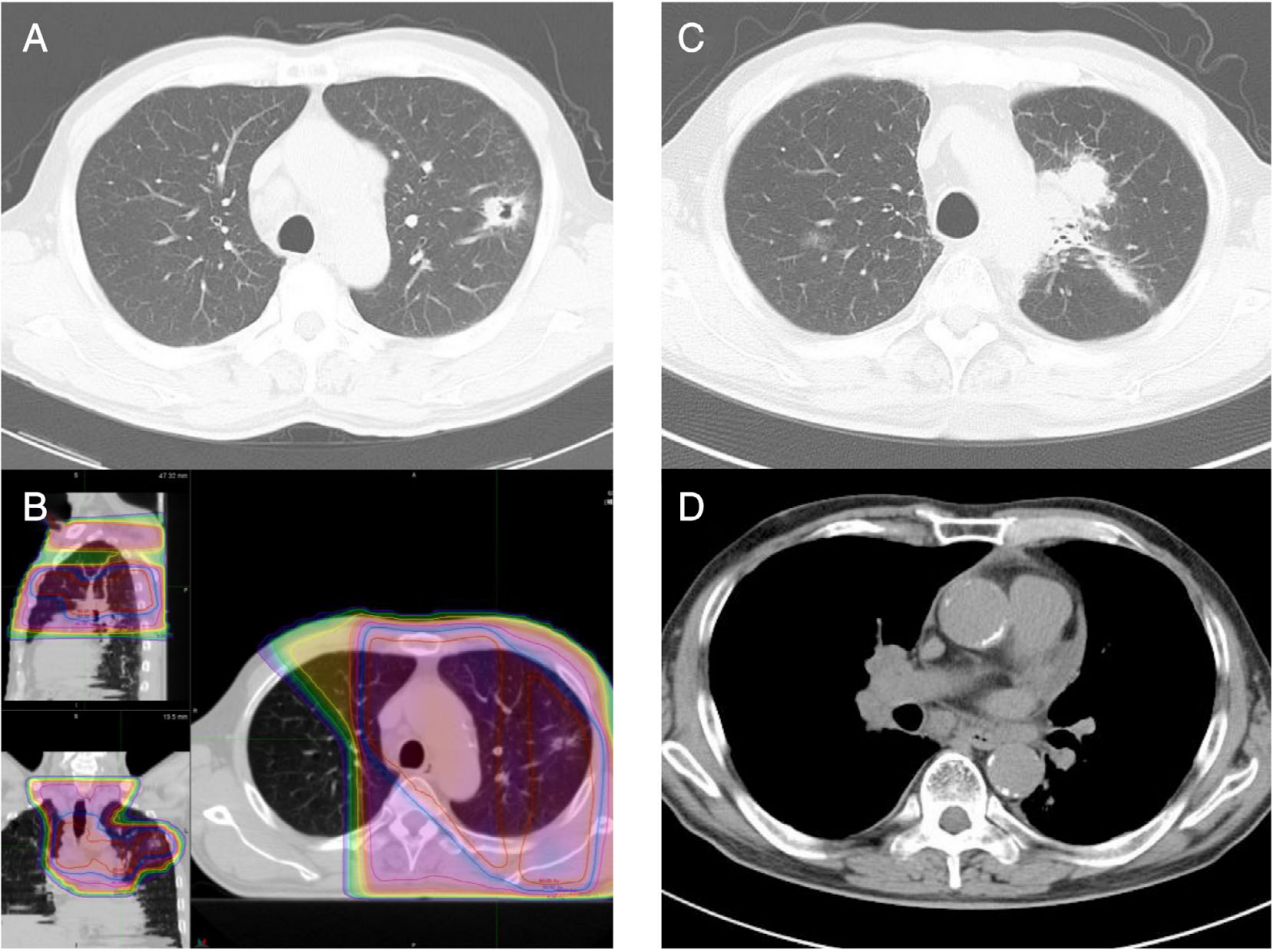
Images of case 1. (A) Thoracic computed tomography showing a pulmonary nodule with a cavity in the left upper lobe and lymph node swelling of the mediastinum at the onset of lung adenocarcinoma when the patient was 62 years. (B) Map of radiation dose distribution in radiotherapy for lung adenocarcinoma. (C) A mass seen in the left upper lobe when the patient visited the hospital for haemoptysis. (D) Swollen lymph nodes in the mediastinum and contralateral pulmonary hilum.
